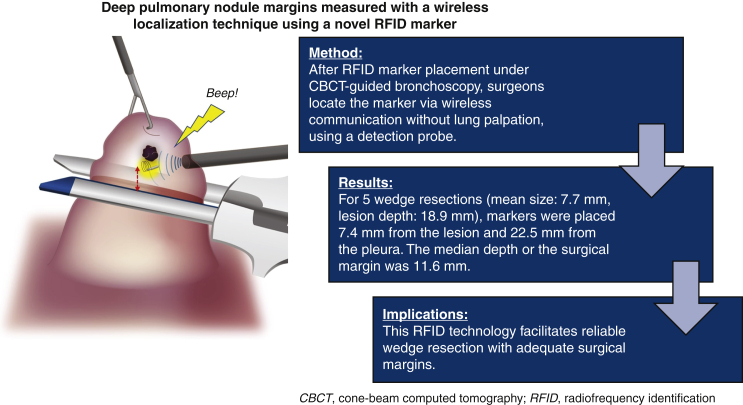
Outline of the wireless localization technique using radiofrequency identification (*RFID*) markers. The deep surgical margin could be measured using a novel RFID marker and wireless communication. *CBCT*, Cone-beam computed tomography.
